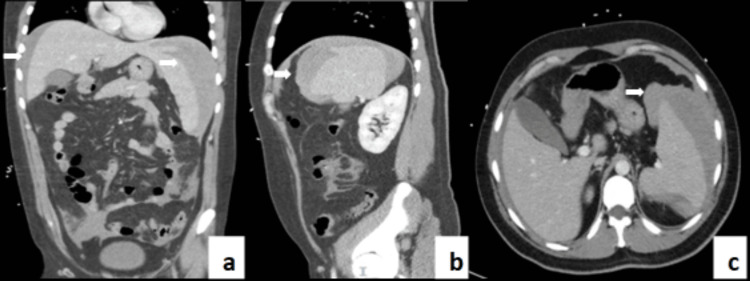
CT images (a. coronal, b. sagittal, c. axial) showing a large volume of hemoperitoneum and the site of splenic rupture White arrows indicate hemoperitoneum. CT, computed tomography
